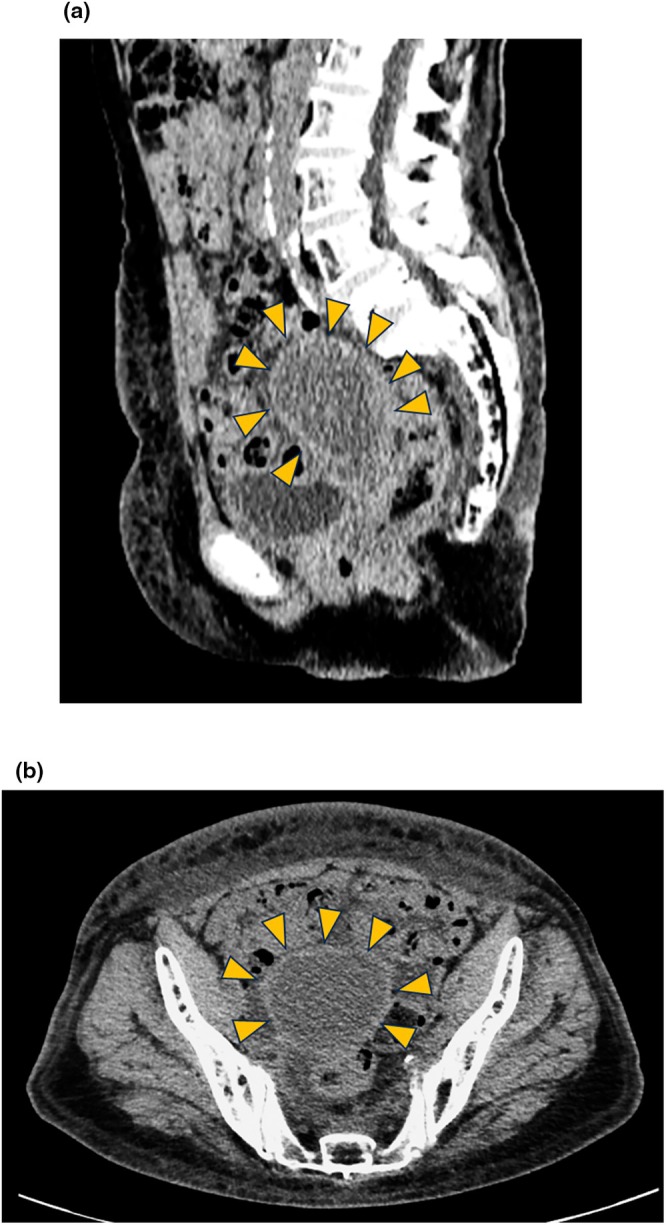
Computed tomography before ethanol sclerotherapy (a) Sagittal section (b) Axial section A large amount of fluid filled the uterine cavity, and the myometrium was thinned. Yellow arrowheads: Outline of the uterine body.
